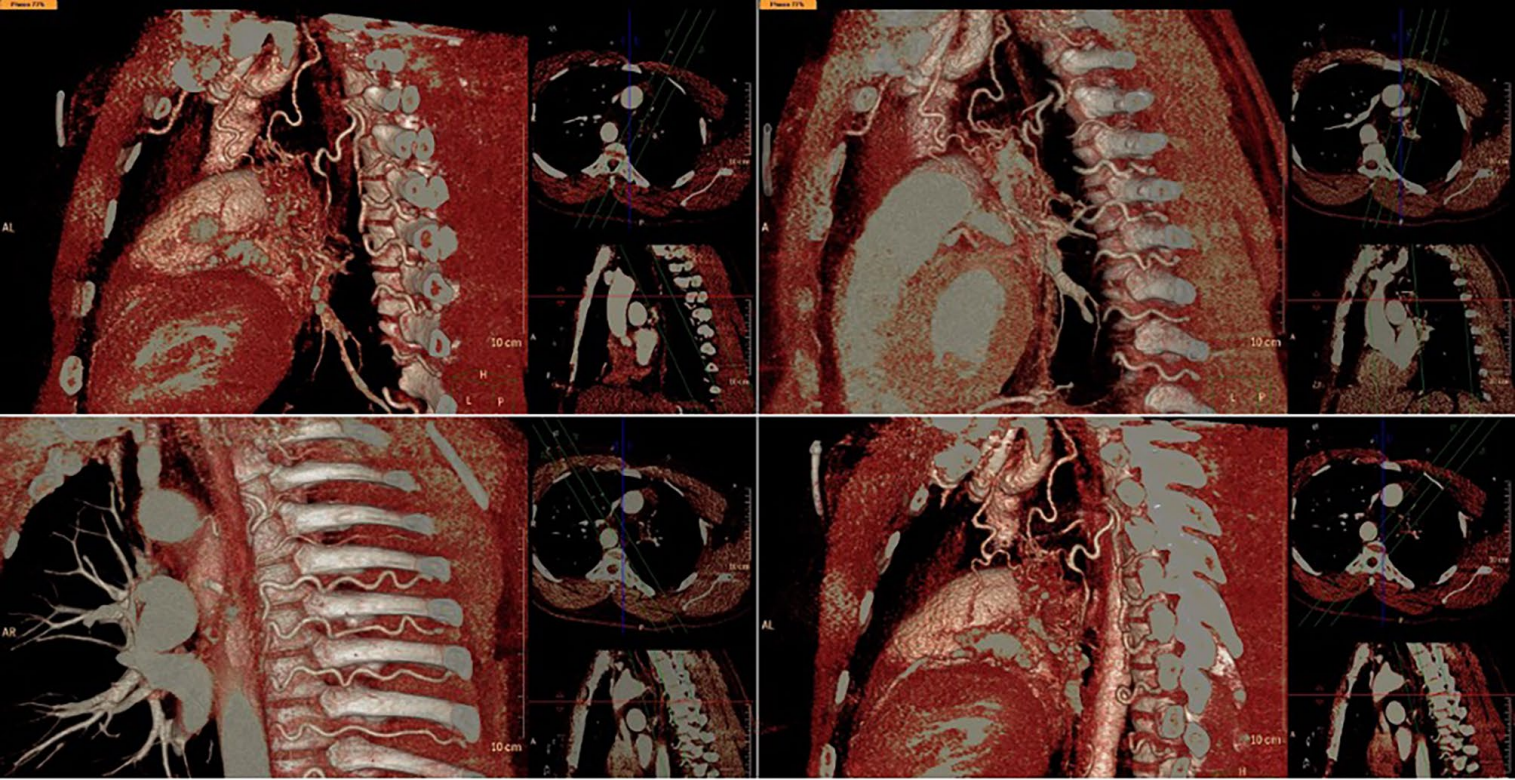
3-D computed tomography imaging reconstruction of the hypertrophied bronchial artery and intercostal arteries
